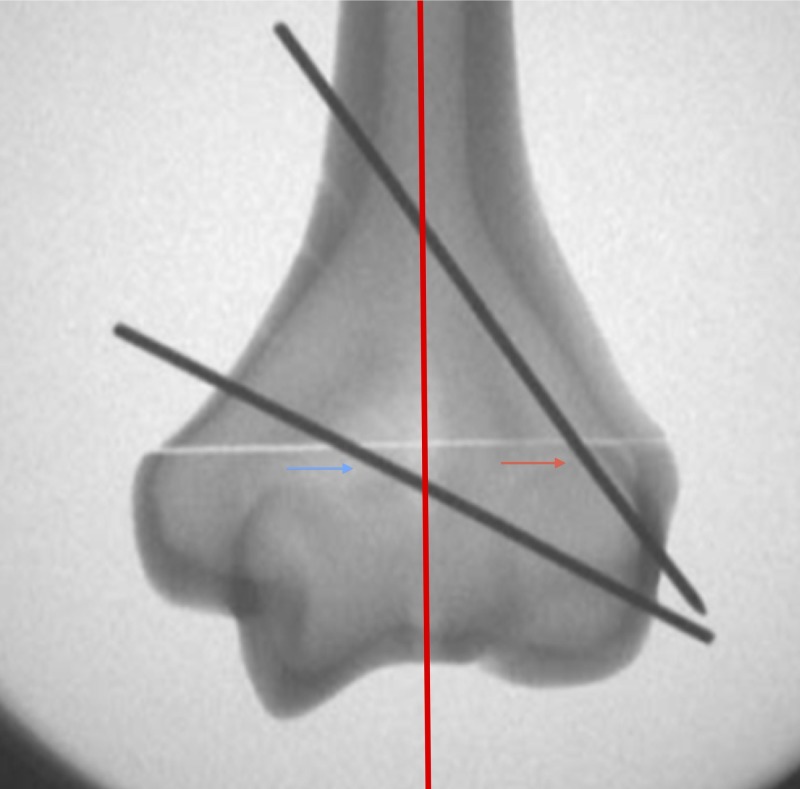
Assessment of AP intraoperative radiograph for bi-columnar fixation. Distal humerus divided into medial and lateral columns (as indicated by the red line). Bi-columnar fixation achieved if (1) a wire is traversing the lateral half of the distal fragment (red arrow); (2) a wire is traversing the medial half of the distal fragment (blue arrow); and (3) both wires have purchase in the medial and lateral cortices. AP: anteroposterior
